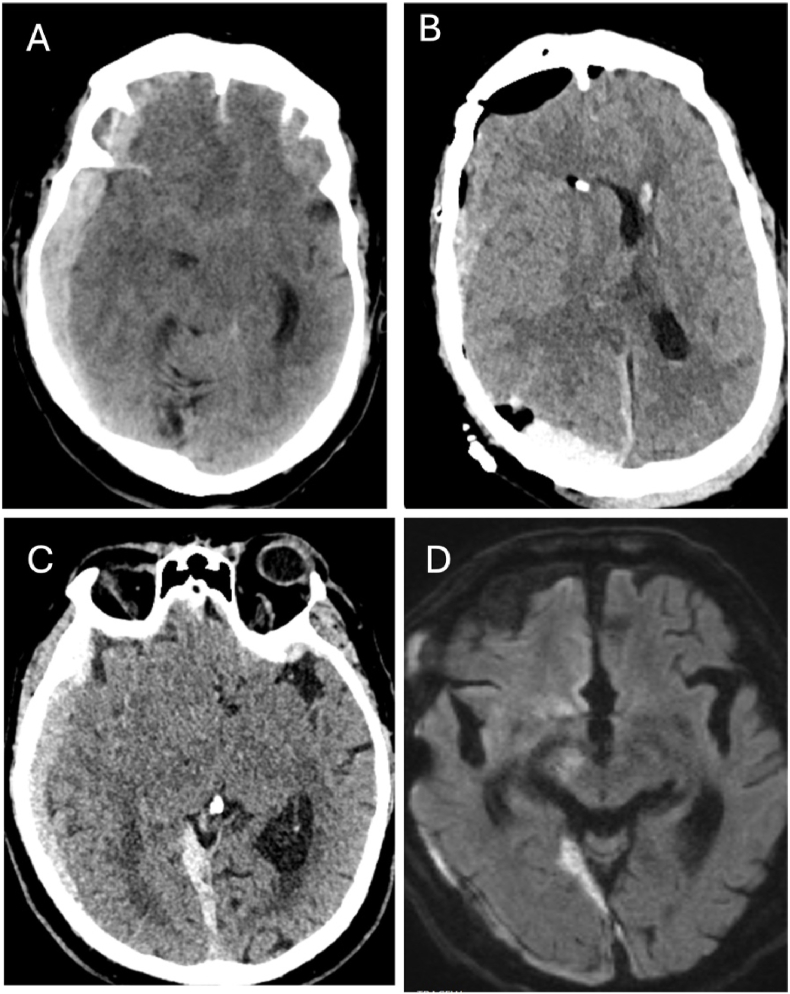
Examples of CT scans of us-TBI patients with a fatal outcome (Glasgow outcome scale extended, GOSE, 1). A-B) A 60-year-old male with a fall accident at work. At the ER he was initially awake, then rapidly deteriorated to GCS 4 with bilaterally dilated but responsive pupils at the local hospital ca 2 h away from our neurosurgical department. Upon arrival, he was immediately taken to surgery for removal of the acute right-sided subdural haematoma. Post-op CT scan (B) shows evacuation of the haematoma although a persistent midline shift and right-sided cerebral swelling was observed. He was then GCS 4 with bilaterally unresponsive pupils. A do-not-resuscitate order was taken on post-op day 2, and step-down care after day 4. He died soon thereafter. C-D) A 76-year-old male on warfarin due to atrial fibrillation fell and deteriorated rapidly to GCS 4 and dilated his right pupil. The warfarin effect was immediately reversed, and the patient was taken to our department where he underwent emergent craniotomy and evacuation of the haematoma. The post-operative CT scan showed a resolution of the midline shift. However, the patient was post-operatively GCS 6 which persisted. An MRI (D) ca 2 weeks post-surgery shows right-sided central infarcts and withdrawal of care was decided on day 20 post-op.
